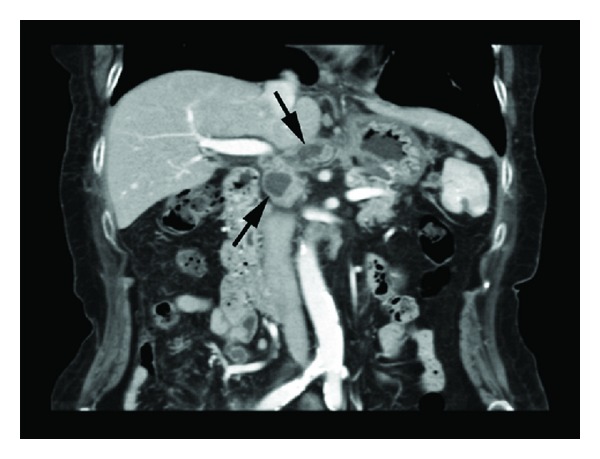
Abdominal computed tomography scan revealed multiple enlarged lymph nodes (arrows).
